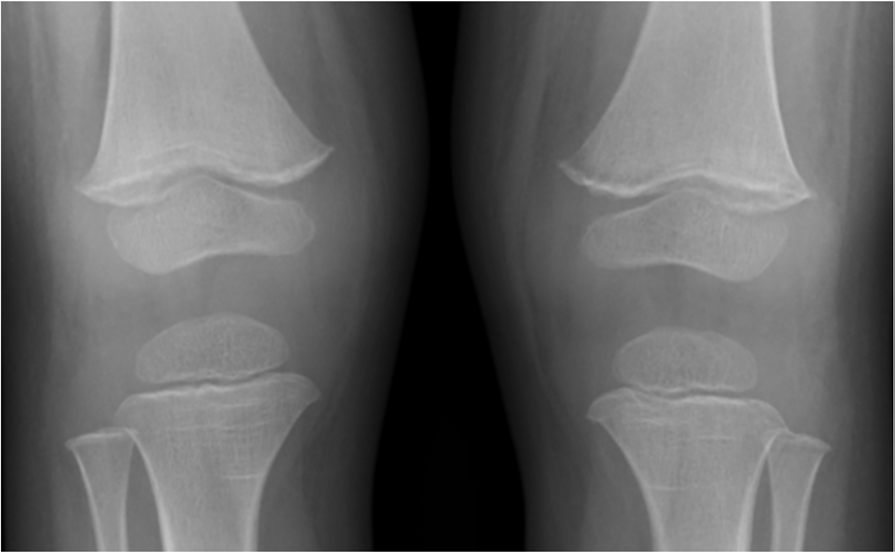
Both knees x-ray showed sclerotic rim at epiphysis
